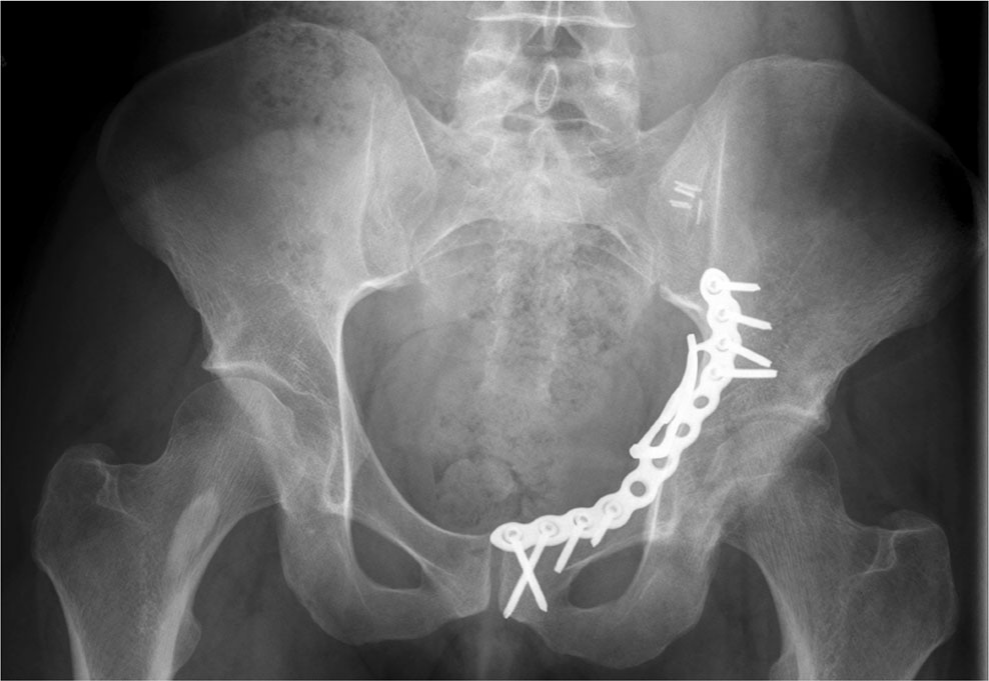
Post-operative X-ray demonstrates the fracture reduced anatomically using a small fragment plate (Stryker PRO system, Stryker Corp., Kalamazoo, MI, USA) on the pelvic brim and quadrilateral plate without any step or gap
